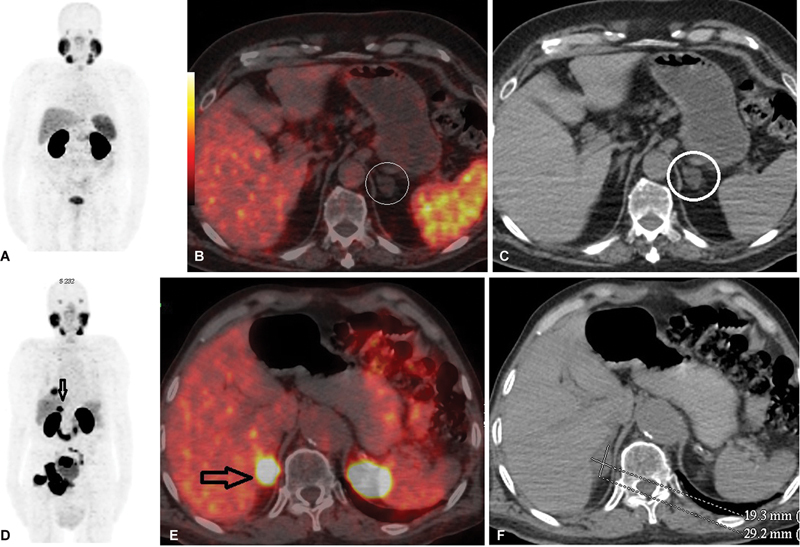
Comparison of PET/CT images showing benign and metastatic adrenal lesion patients. Panels (
